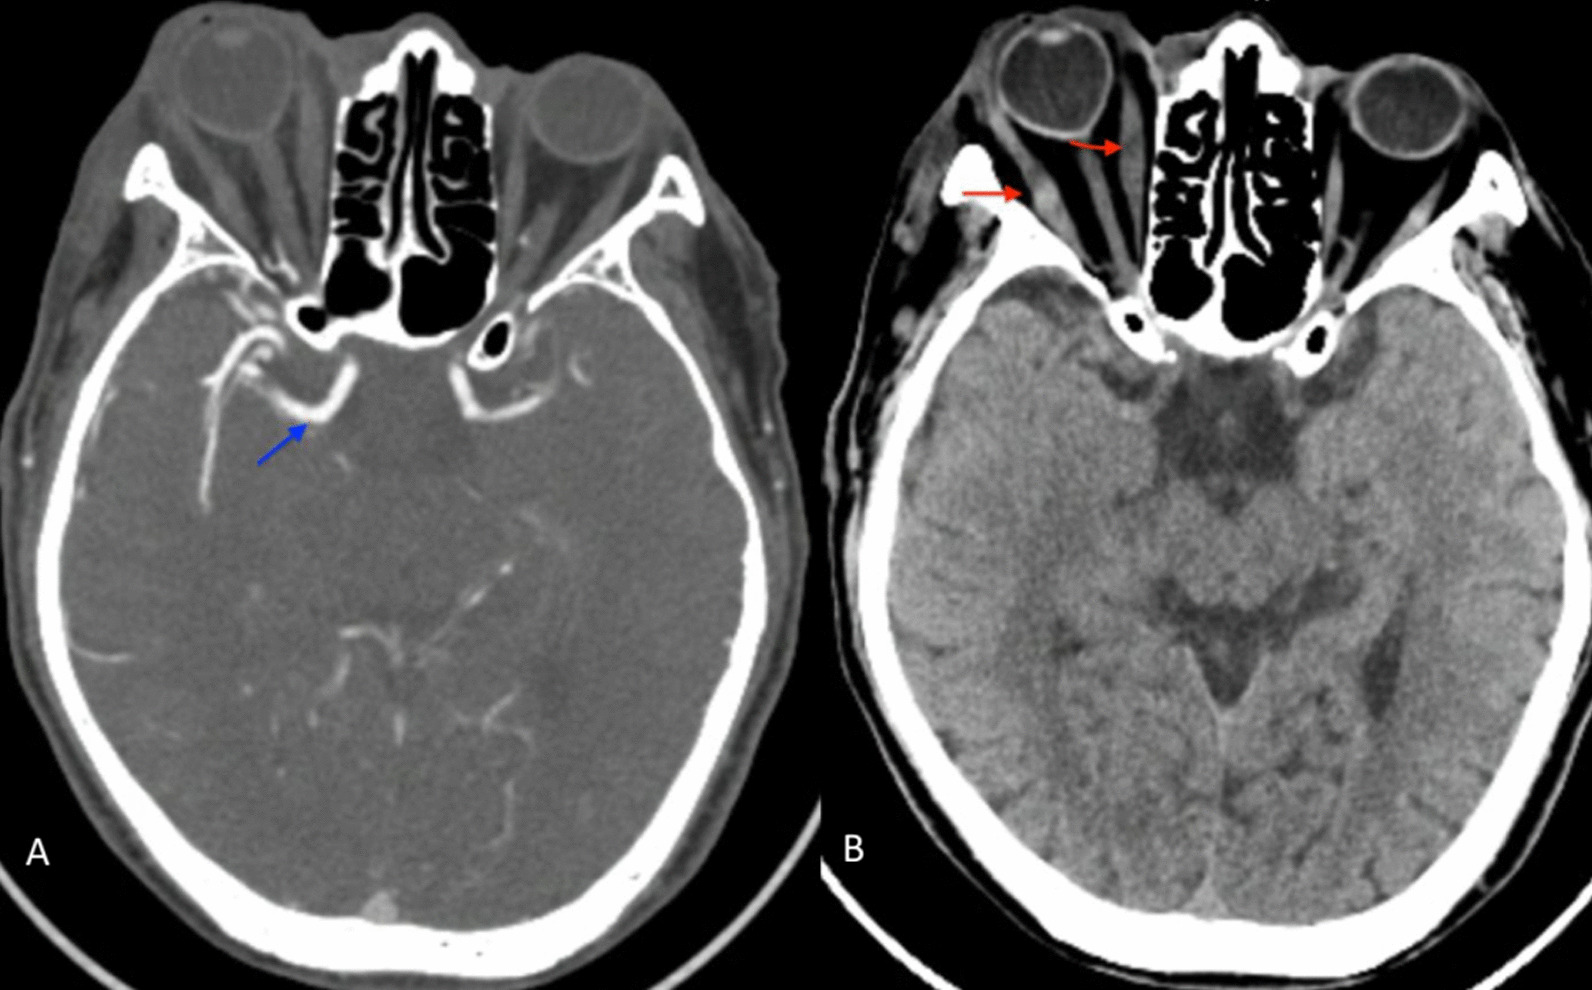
CTA after MT revealing no signs of carotid–cavernous fistula (blue arrow) (A). Slight enlargement of the right medial and lateral rectus muscles (red arrows) (B)
